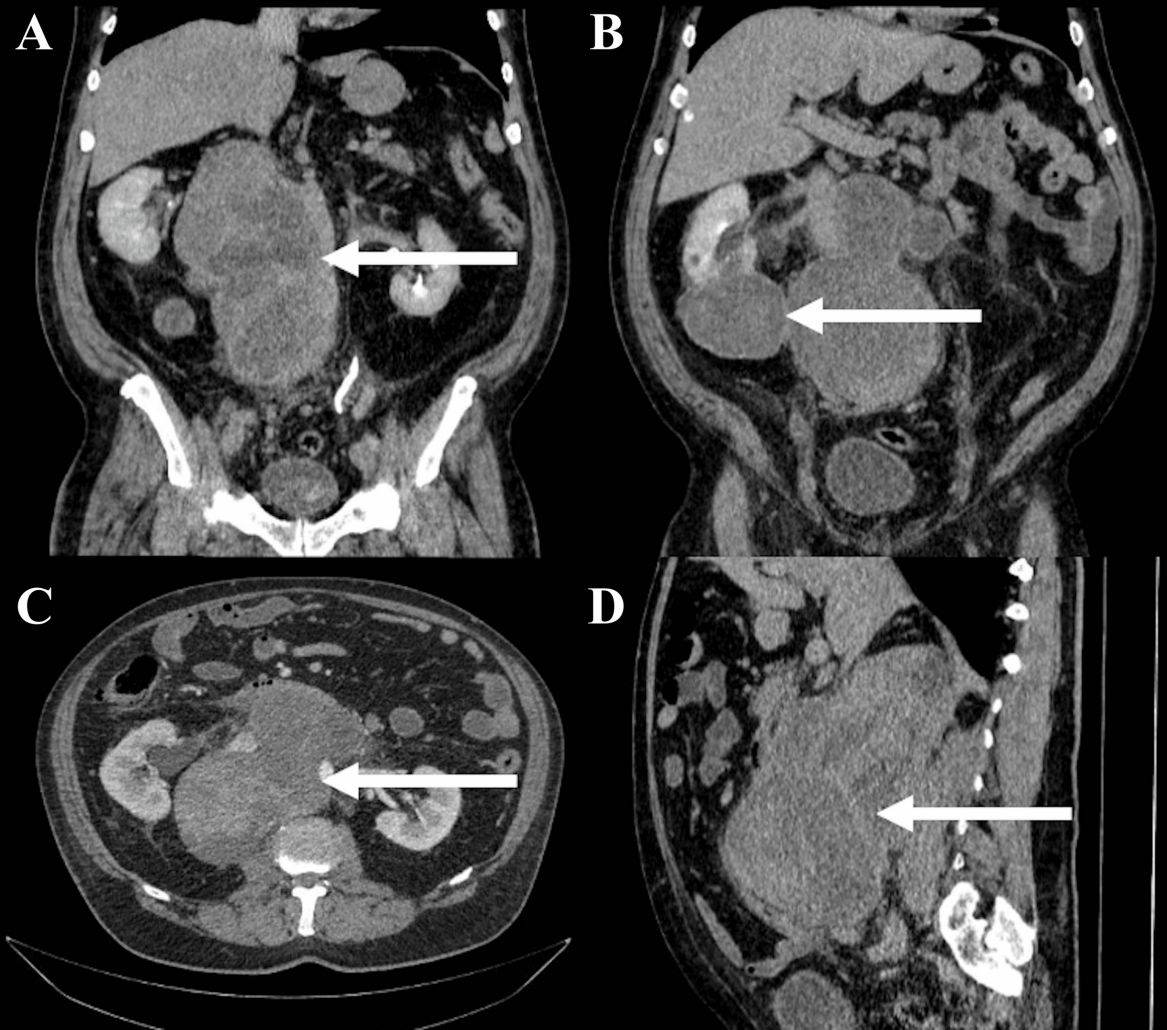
CT of the abdomen and pelvis in coronal (A and B), axial (C), and sagittal (D) planes The images show a large pelvic mass measuring 224x 162 x 111 mm in the longitudinal, transverse, and anteroposterior dimensions, respectively. It completely compresses the inferior vena cava immediately proximal to the inferior vena cava conformation, wraps around the abdominal aorta below the renal arteries, displacing the right renal artery (white arrows A, C, D). There is also a right renal mass in the lower pole measuring 84 x 76 mm (white arrow B) CT: computed tomography
